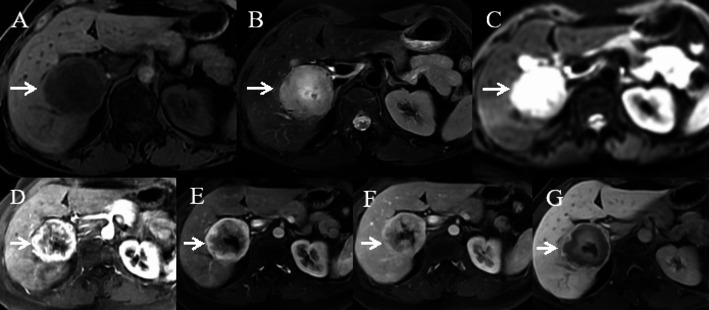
Gd-EOB-DTPA MRI of the lesion. A, B Long T1 and mildly prolonged T2 signals. C Restricted diffusion on DWI. D, E Arterial and portal phases showing heterogeneous ring enhancement and partial washout, suggestive of HCC. F Delayed phase central enhancement. G Low hepatobiliary phase signal, consistent with initial misdiagnosis
